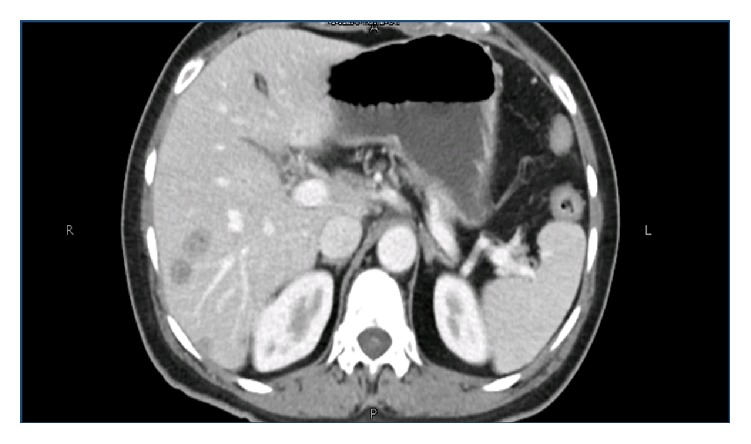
CT abdomen with contrast: multiple hypoattenuating lesions in the liver.
